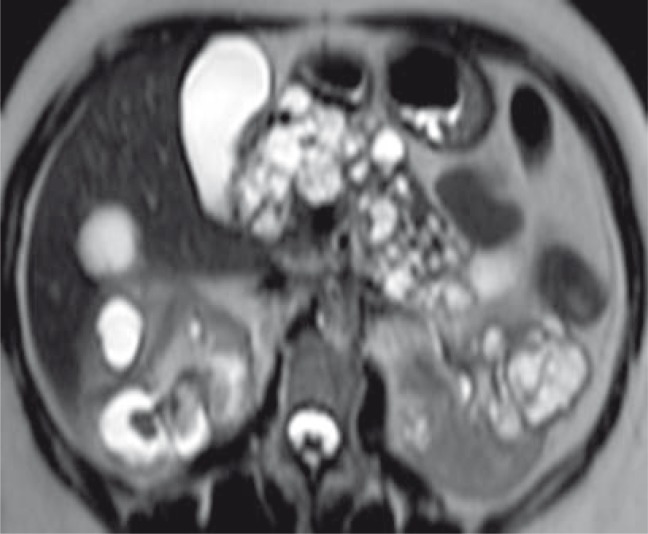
Axial T2 weighted MR image shows multiple cysts in kidney and pancreas.
